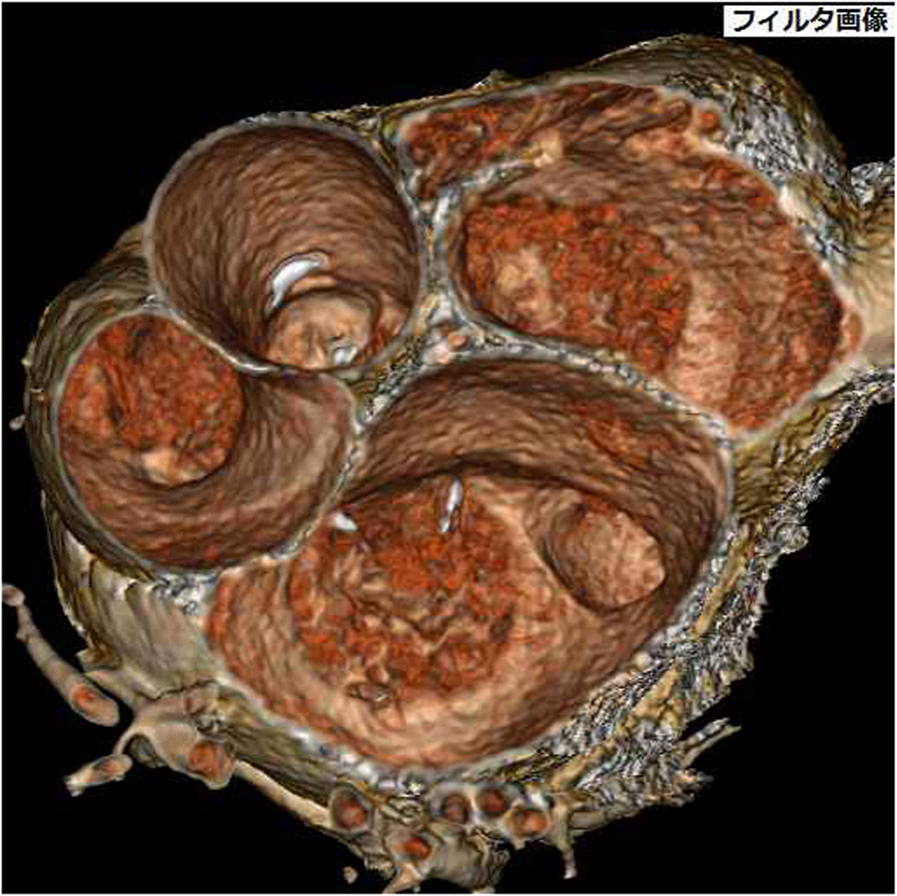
Sixty-four-row multidetector computed tomography in case 5. Sixty-four-row multidetector computed tomography in case 5 showing the morphology of the patient’s congenital heart disease. The arrow indicates the coronary sinus defect. *Ao* aorta, *MV* mitral valve, *PA* pulmonary artery, *TV* tricuspid valve
